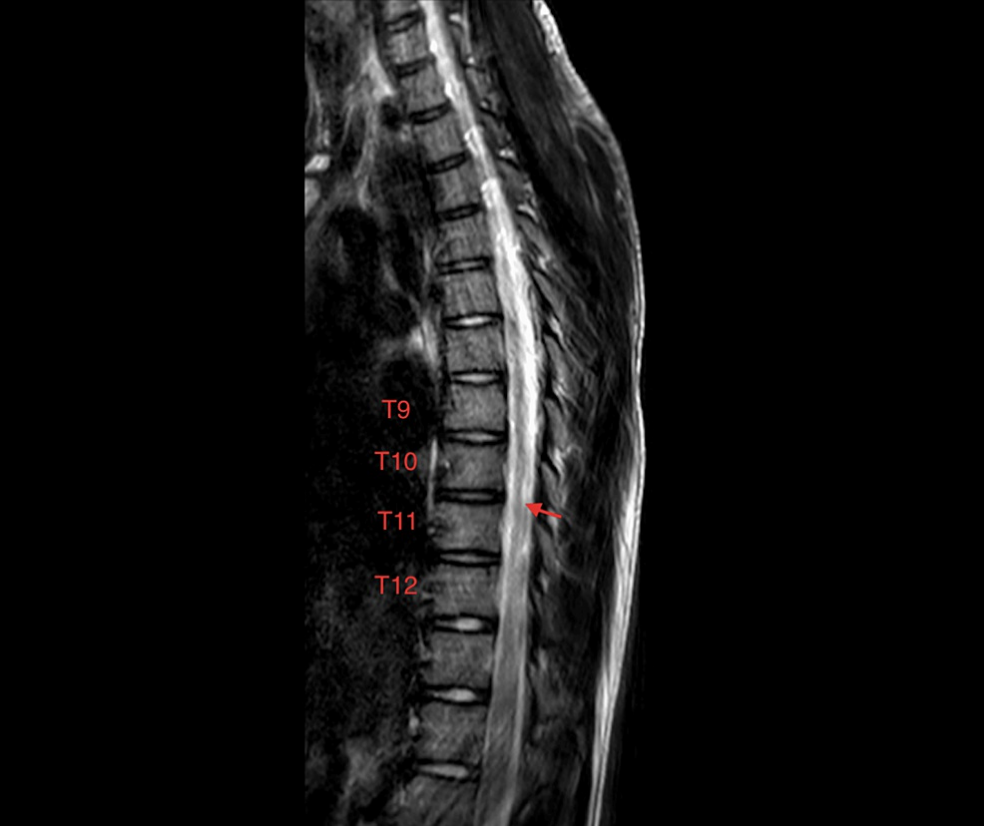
T2 weighted sagittal MRI images demonstrate hyperintense signal in the distal thoracic cord from T9-T12
